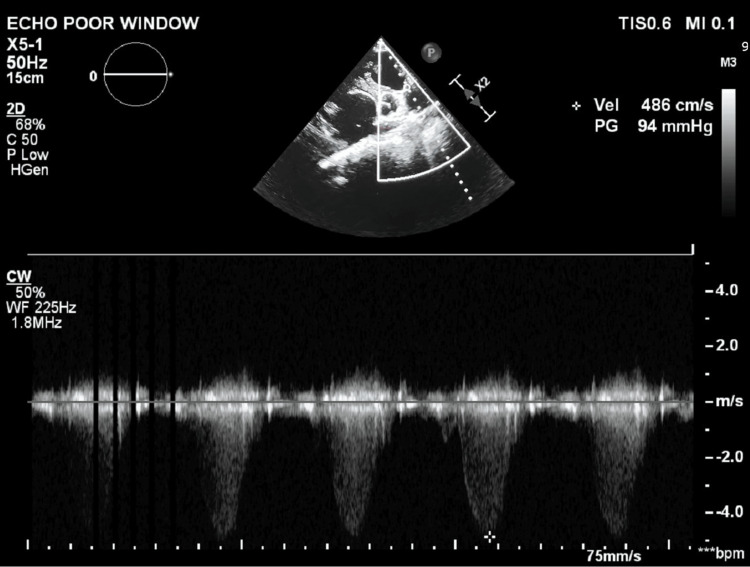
Transthoracic echocardiography (ECHO) in the parasternal short-axis view with continuous wave Doppler across the pulmonary valve, demonstrating severe valvular pulmonary stenosis with a pressure gradient of 94 mmHg.
